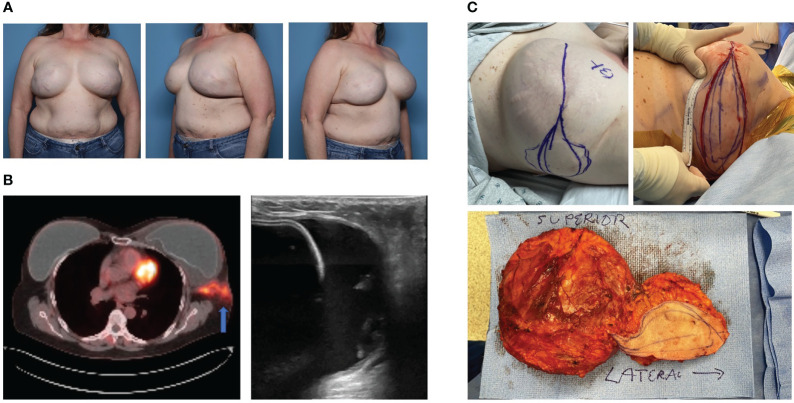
Case of Bilateral Disseminated BIA-ALCL. (A) A 52-year-old female with history of left stage 1 breast cancer and right ductal carcinoma *in situ* who underwent neoadjuvant chemotherapy, bilateral mastectomy and implant based breast reconstruction (subpectoral macrotextured breast implants) in 2008. She developed left breast swelling in 2021 and seroma aspirate was consistent with BIA-ALCL. (B) PET-CT revealed increased metabolic activity within the left capsule with associated extracapsular involvement into the ipsilateral axilla. Ultrasound revealed intracapsular fluid collection surrounding the implant. (C) Bilateral *en bloc* capsulectomies were performed with mass resection extending to the left axilla. Pathology revealed left Stage 4 BIA-ALCL and incidentally found right Stage 1 BIA-ALCL. The patient underwent adjuvant left chest wall radiation and brentuximab vedotin immunotherapy. The patient has had a complete metabolic response to therapy and no evidence of recurrence at 20 months post-operatively.
